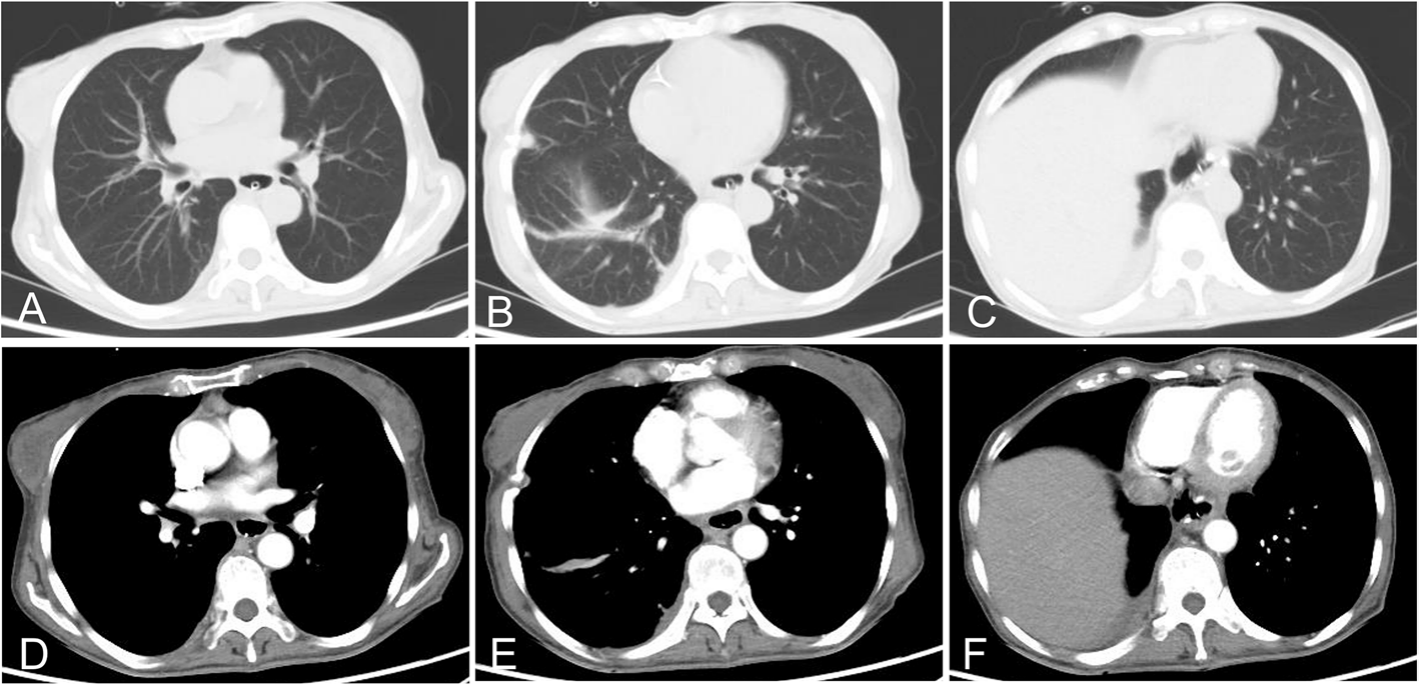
Two months after the intervention, CT scan shows that the accumulated fluid in the lower lobes of both lungs has disappeared, and that the inflammation of the lungs has improved considerably
